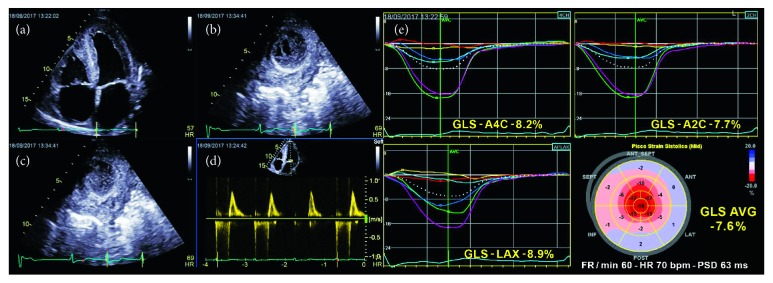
Clinical case of a 47-year-old man with amyloidosis. Please note left ventricular hypertrophy with paracardiac pathological tissue (Panels (a)-(c)) and diastolic dysfunction as assessed by mitral peak early (e) and late (a) velocities restrictive pattern (E/A > 2) with lowest, almost zero, A wave amplitude suggestive of atrial impairment (Panel (d)). Panel (e) shows left ventricular two-dimensional speckle-tracking echocardiography longitudinal strain curves and bull's-eye map with typical “apical sparing” pattern (Panel (e)).
